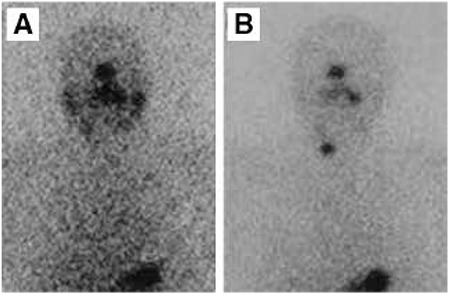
Depicted post-therapeutic and follow up I-131 whole-body scintigraphy images of a 41-year old female patient operated for papillary thyroid cancer and received 150 mCi radioactive iodine treatment. A) I-131 whole-body scintigraphy performed at 6 months post-radioactive iodine treatment showing no activity in the cervical region consistent with ablation,B) I-131 whole-body scintigraphy of the same patient performed 5 years after radioactive iodine treatment revealing increased focal activity in the right lobe of thyroid consistent with recurrent malignancy (stimulated serum thyroglobulin level of the patient during this period was 1.02 ng/dl)
